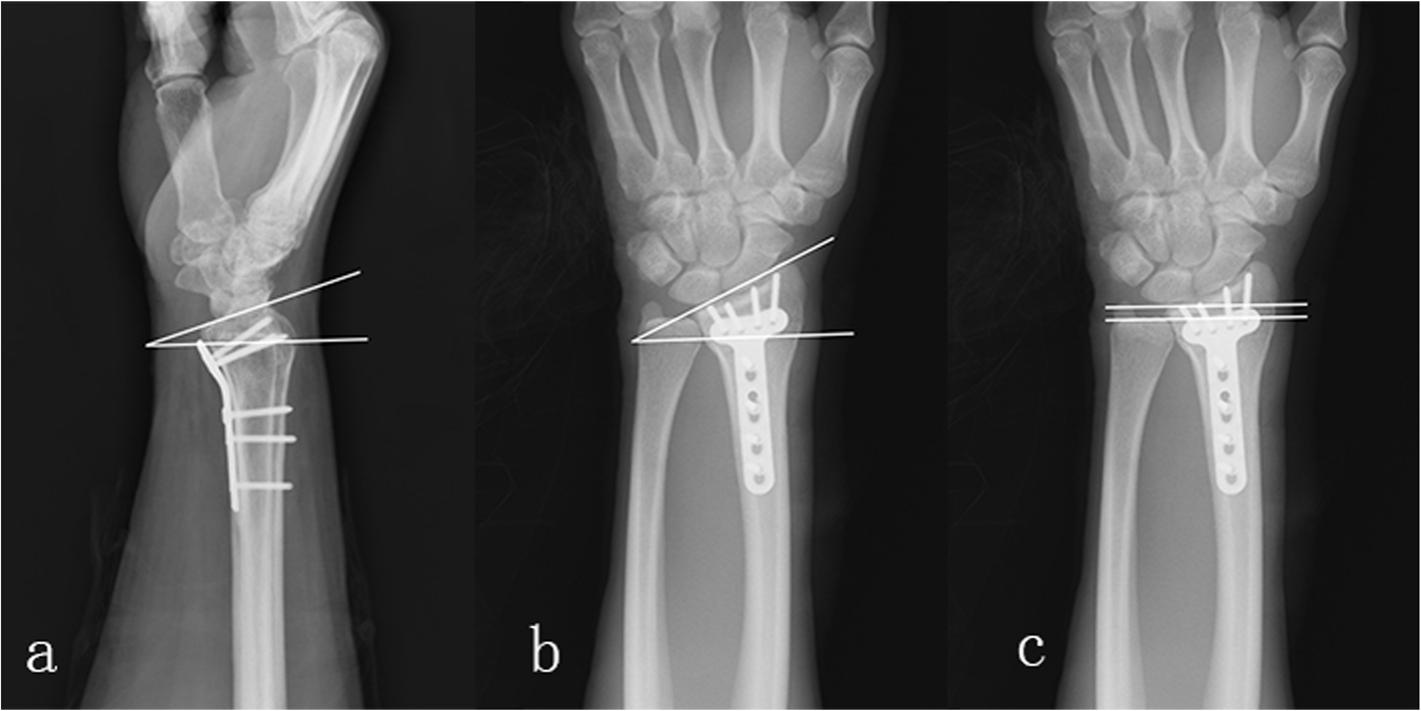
Postoperative x-ray film showing the measurement of radiographic parameters. a Volar tilt was the angle between the line along the distal radial articular surface and the line perpendicular to the longitudinal axis of the radius at the joint margin. b Radial inclination was the angle formed between the long axis of the radius and a line drawn from the distal tip of the radial styloid to the ulnar corner of the lunate fossa. c We identified the long axis of the radius and drew a line perpendicular to this, extending through the ulnar-most corner of the lunate fossa. Ulnar variance was the distance between this line, and the distal-most point of the ulnar dome was recorded as the ulnar variance
